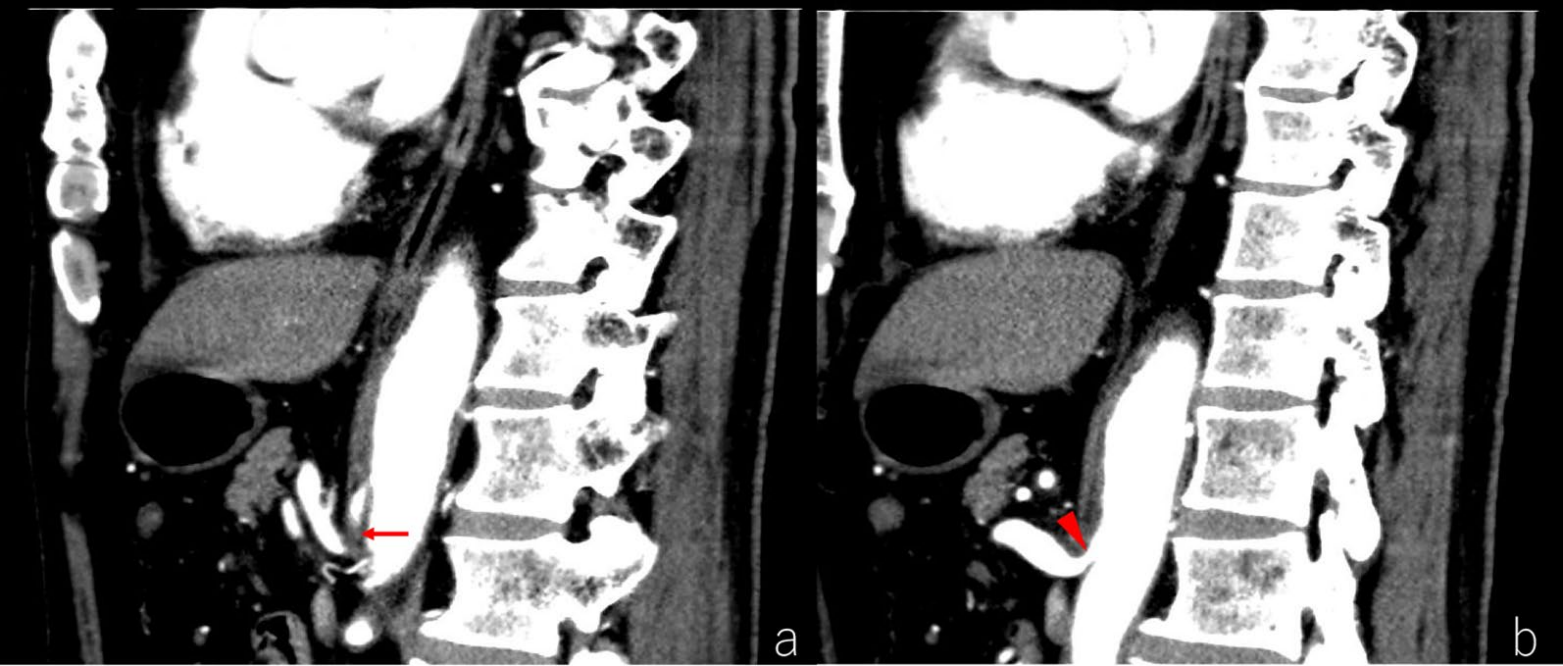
Enhanced CT findings (sagittal). a The arterial phase of enhanced CT reveals the almost occluded celiac arterial root (red arrow) and post-stenotic dilatation. b Arterial-phase enhanced CT displays mild stenosis at the root of the superior mesenteric artery (red arrowhead)
